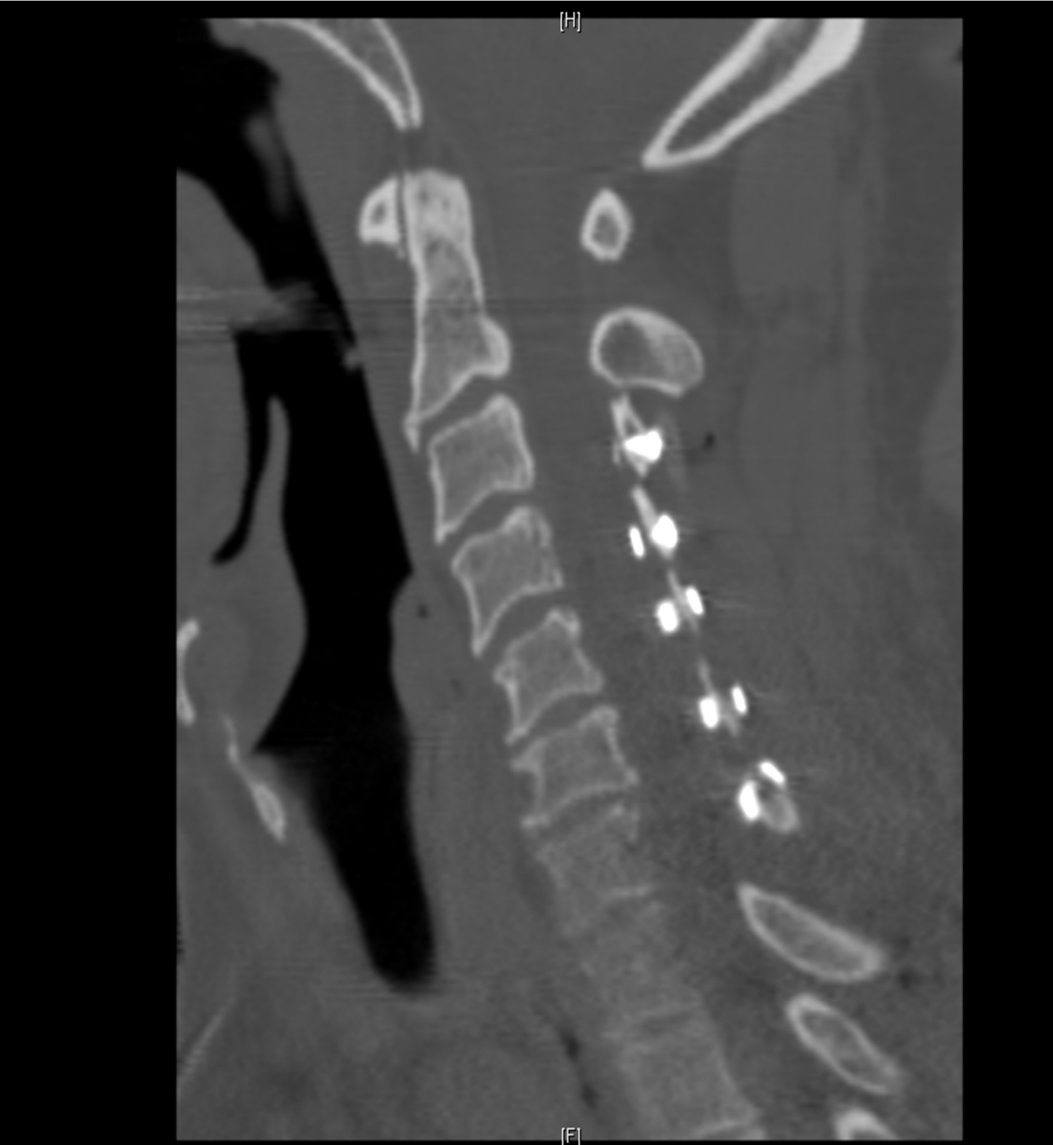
CT (sagittal view) of cervical region showing normal post-operative changes.
